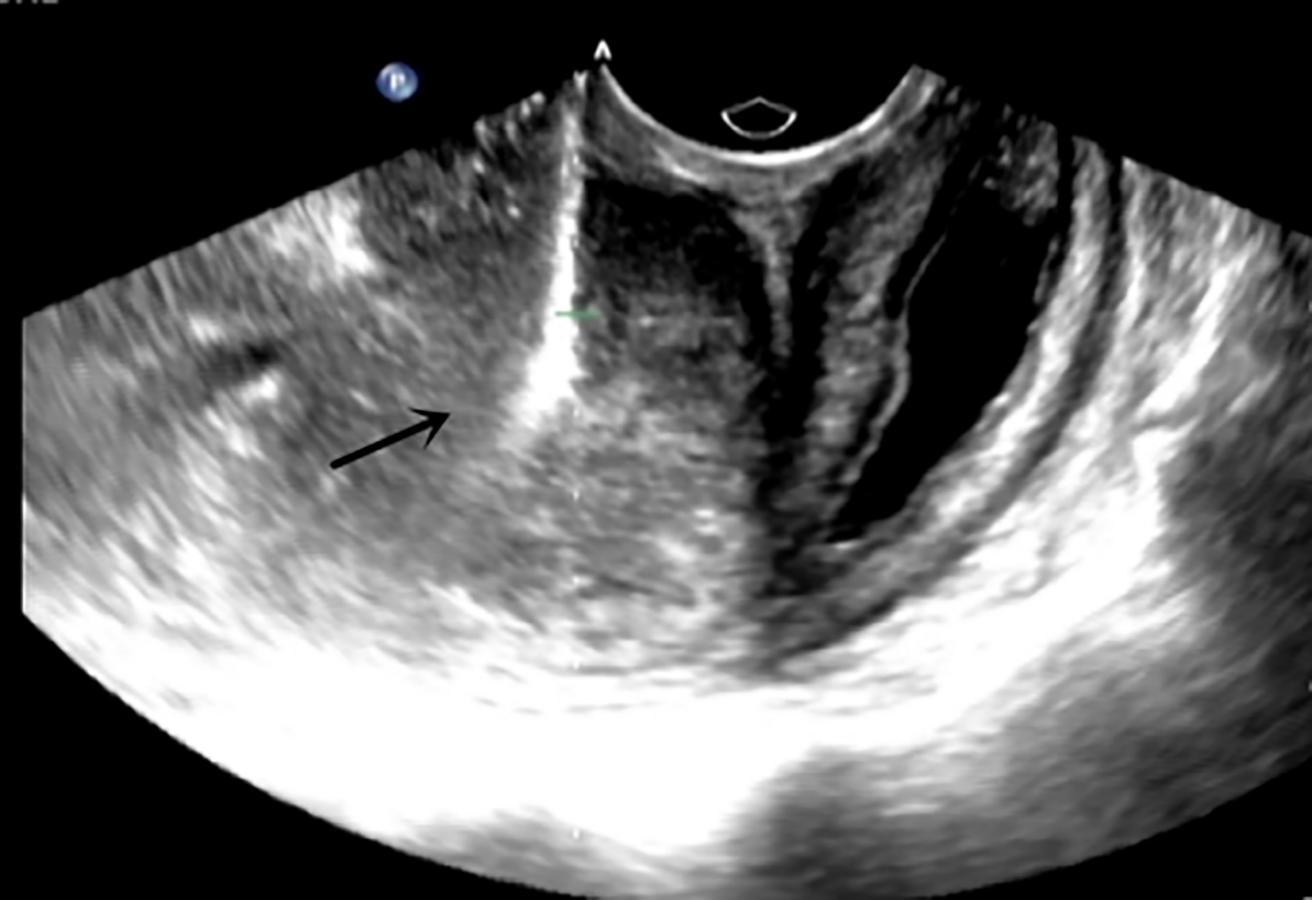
Ultrasound guided transvaginal pelvic mass biopsy.
